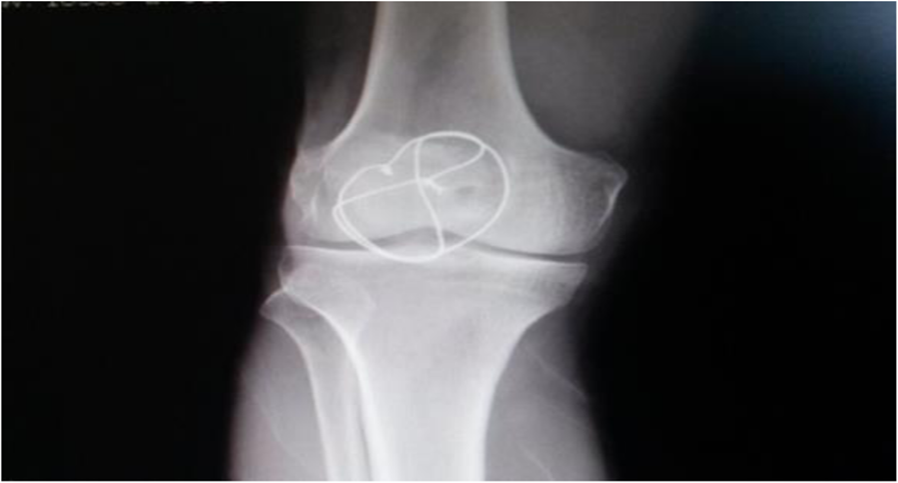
Anteroposterior plain radiography showing a cerclage wire from the tension band fixation of the patella of the ipsilateral knee
